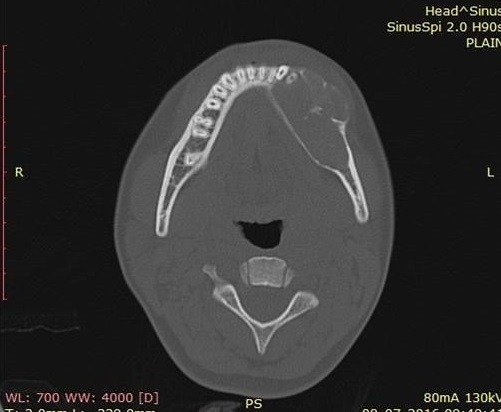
Computed tomography view
